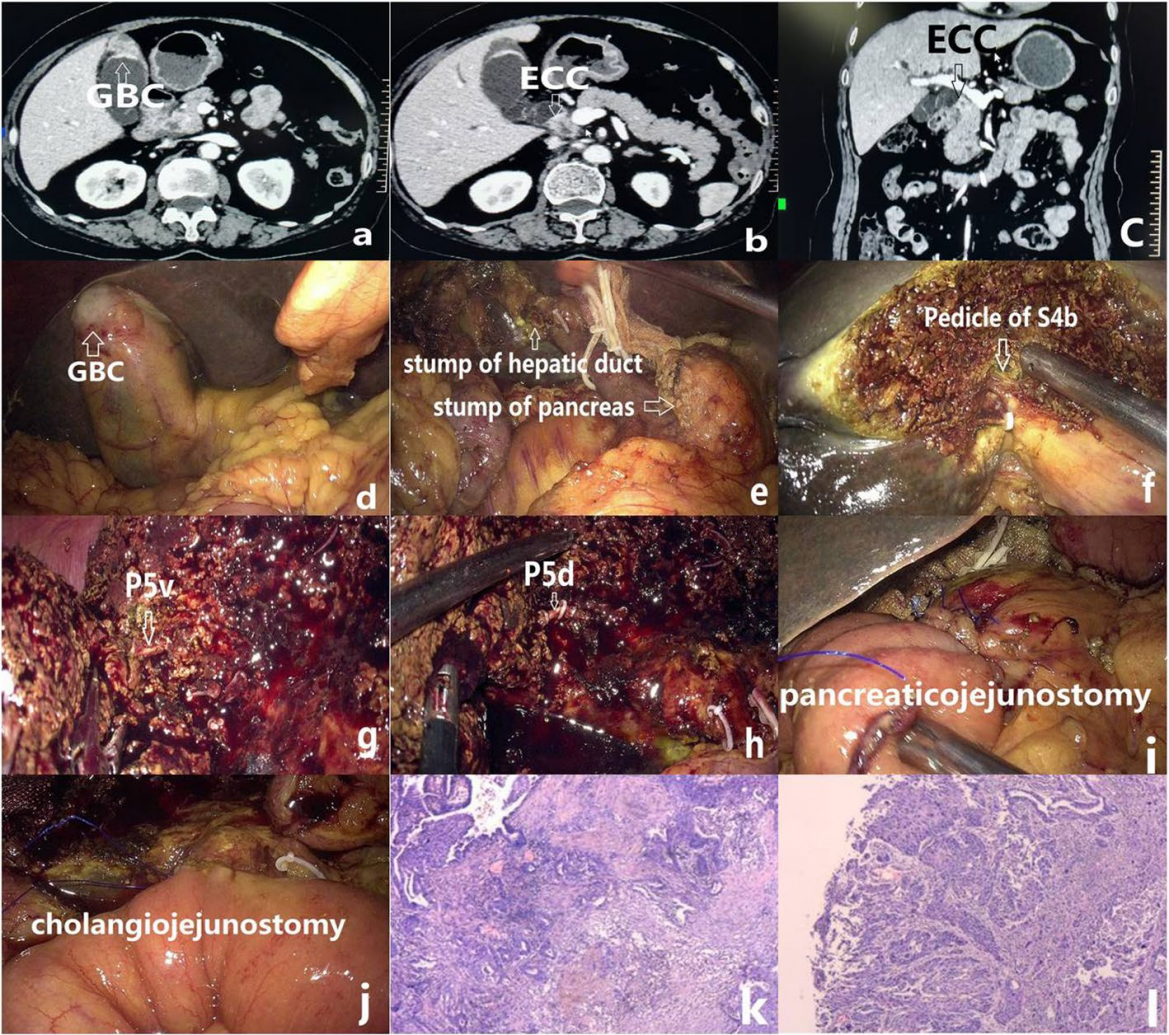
a Thickened wall of gallbladder by enhanced CT. b Thickened wall of common bile duct by enhanced CT. c Thickened wall of common bile duct by enhanced CT. d Suspected gallbladder cancer during operation. e Stump of the pancreas and hepatic duct. f Hepatic pedicle of segment IVb. g Ventral portal vein of segment V. h Dorsal portal vein of segment V. i Pancreaticojejunostomy. j Cholangiojejunostomy. k Pathology of gallbladder (× 100). l pathology of a common bile duct (× 100)
